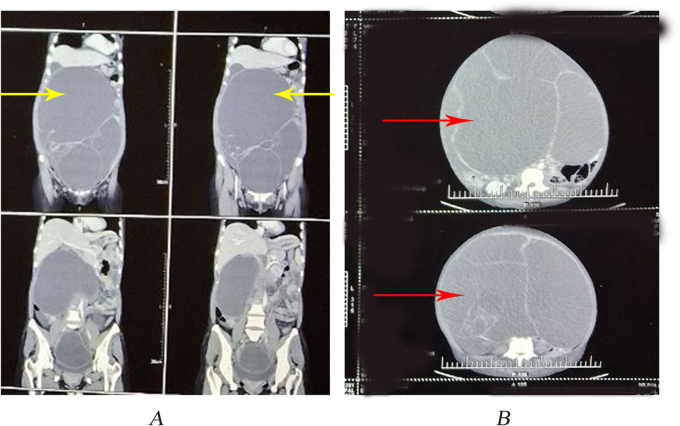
Contrast-enhanced CT of the abdomen and pelvis. (A) Coronal view showing a giant multiloculated cystic abdominopelvic mass (yellow arrows). (B) Axial view demonstrating internal septations and peripheral solid components (red arrows), with compression of surrounding abdominal organs.
